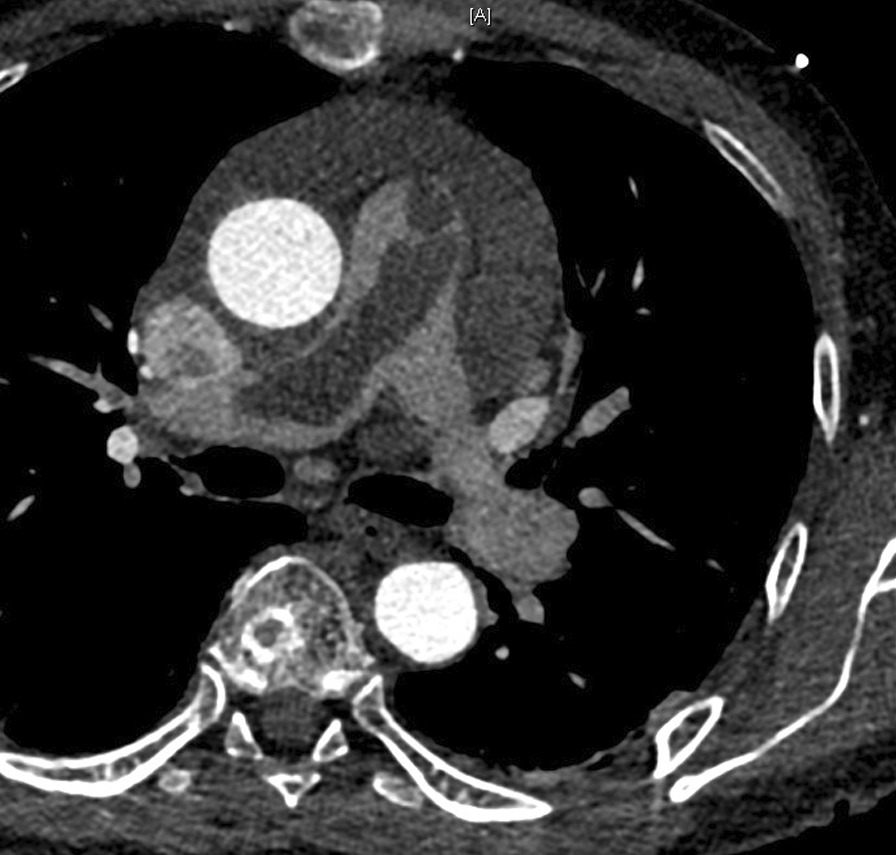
Preoperative CT-scan showing the tumor in the main and right pulmonary arteries
